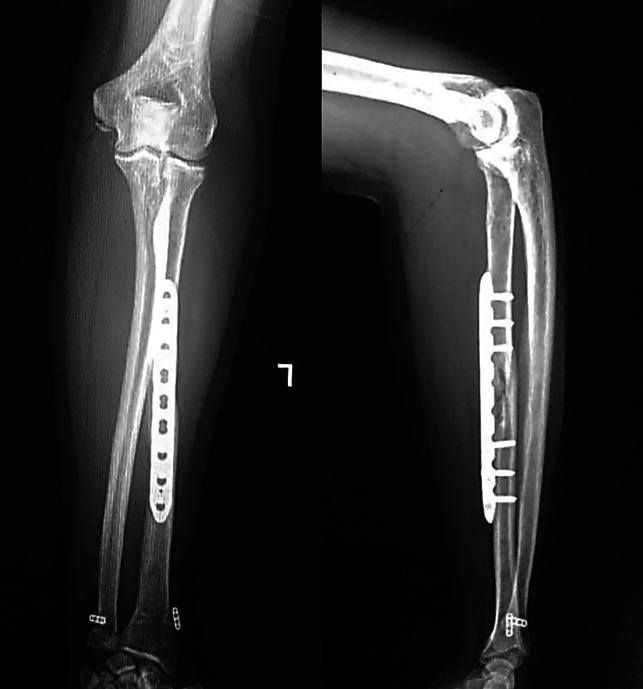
Eighteen-month follow-up radiographs showing anteroposterior and lateral views.
